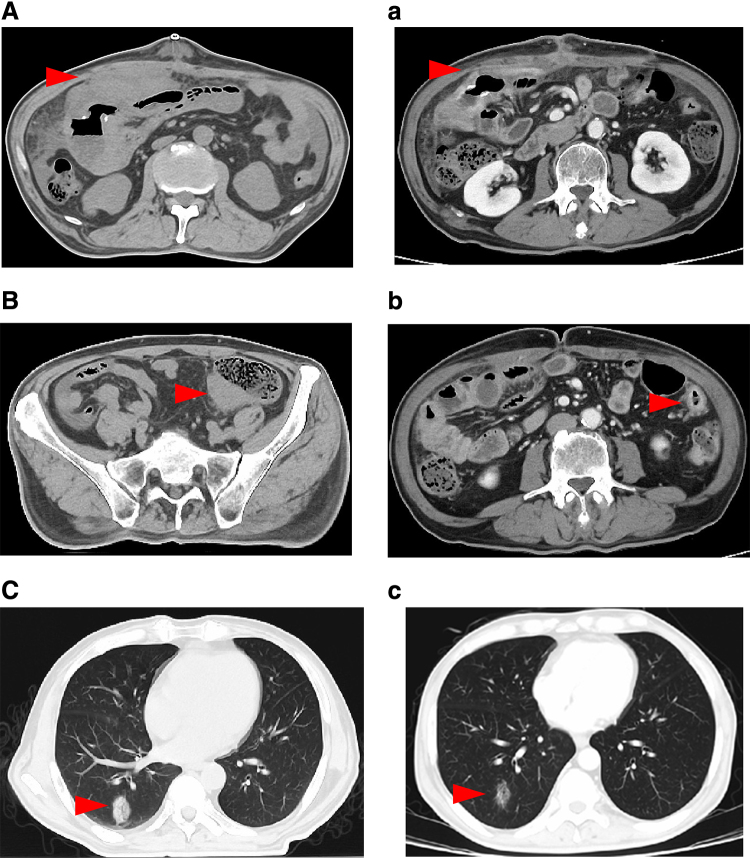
CT before RT (A–C) and contrast-enhanced CT just after RT (a–c). The red arrows indicate each individual lesion. A/a, small bowel; B/b, sigmoid; C/c, lower lobe of the right lung.
